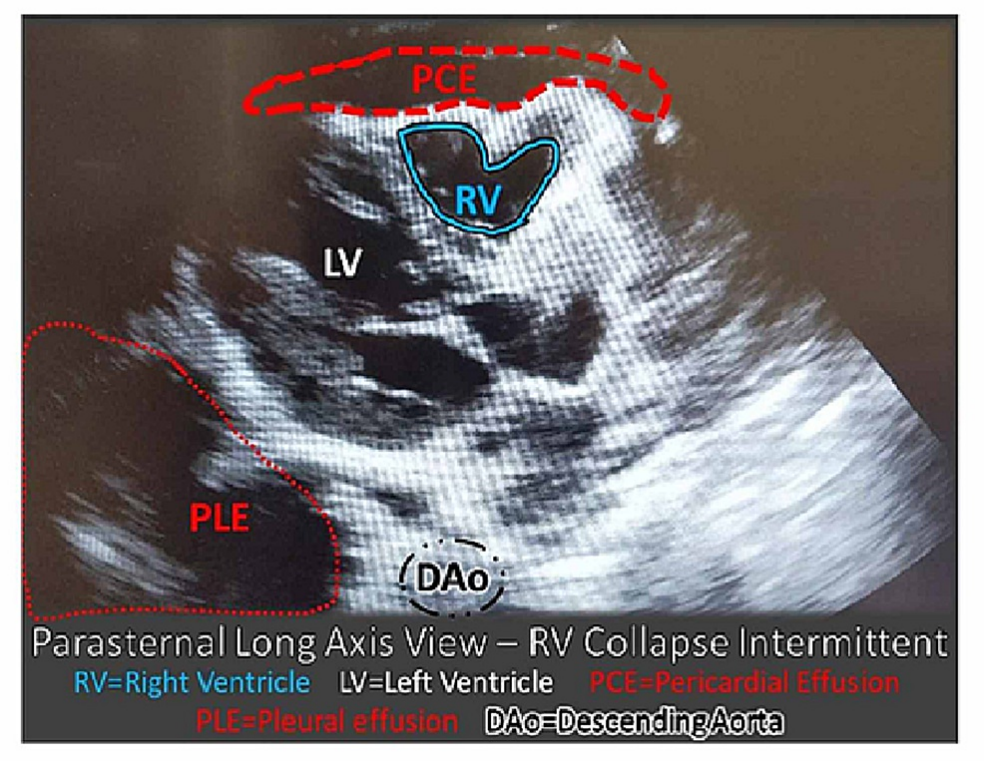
POCUS of the heart parasternal long-axis view – note the RV is collapsing in diastole. There is a small PCE. A large PLE is noted. Right ventricle collapse is not in proportion to the size of the pericardial effusion. When considered in the context of the pleural effusion, it is suggestive of a probable mass effect. POCUS: point-of-care ultrasound; PCE: pericardial effusion; PLE: large pleural effusion; DAo: descending aorta; RV: right ventricle
